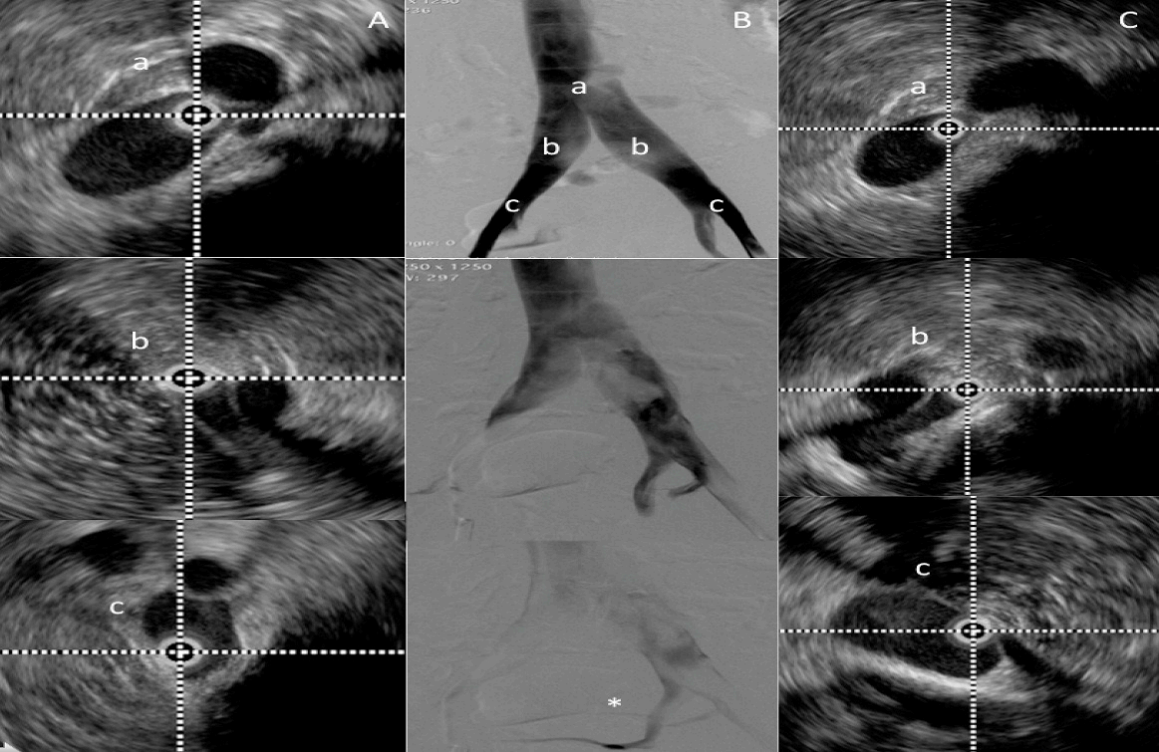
Venography and intravascular ultrasound for determination of degree of obstruction and segment obstructed: (A) Right limb; (B) Venography; (C) Left limb; *Confluence of the internal and external iliac veins; b- Common iliac vein; c- Confluence of the external and internal iliac veins.
